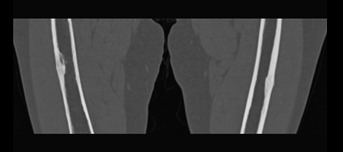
CT Scan Showing Bilateral Lateral Cortical Thickening.
